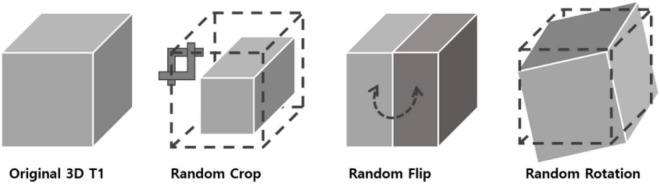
3D data transformations used for data augmentation. Three types of geometrical transformations were applied to the 3D MRI data augment the data set: cropping of the image, left-right flips, and in-plane rotations.
